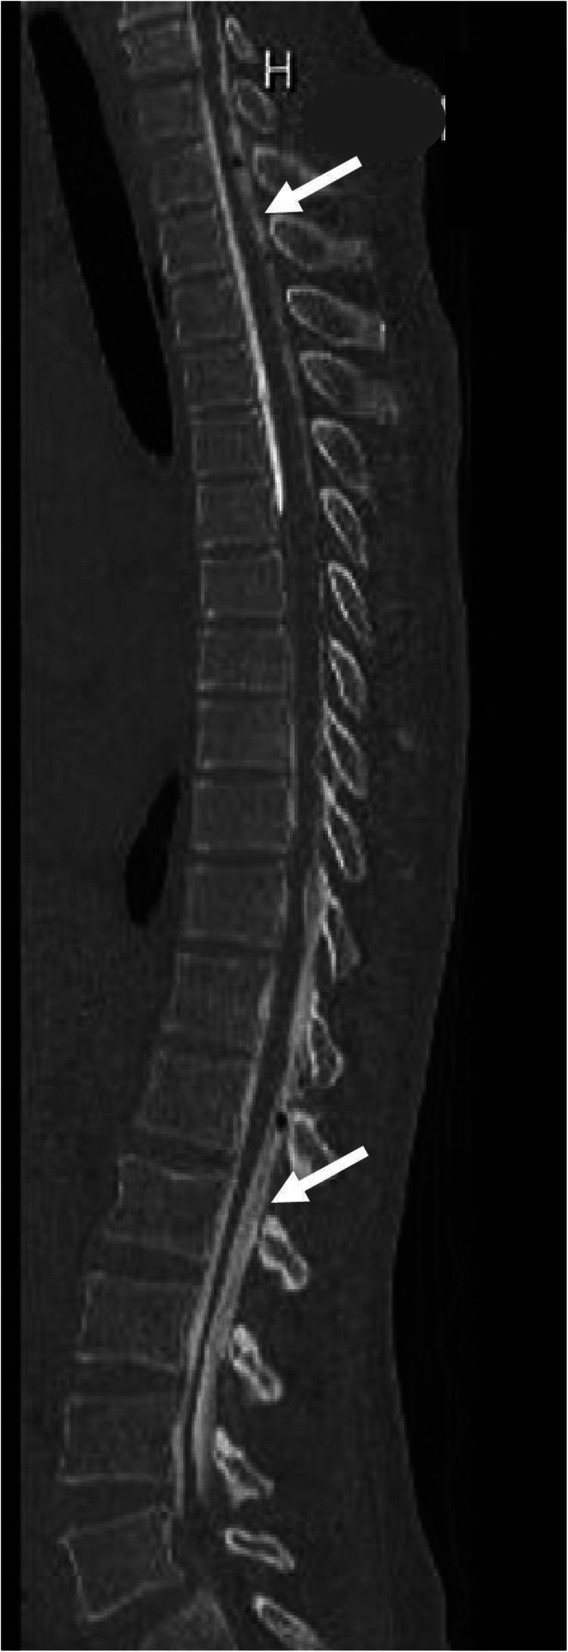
Sagittal cervicothoracic spinal MRI showing hyperintense epidural fluid collection (arrows) indicating sealing of the leakage site. MRI, magnetic resonance imaging
